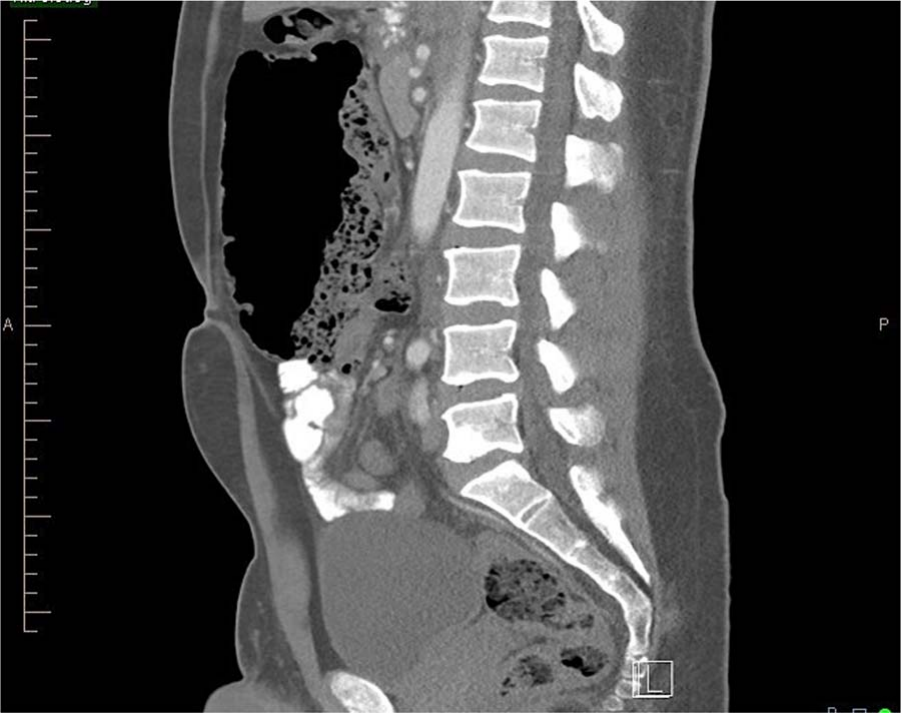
Sagittal view of CT abdomen showing higher and more anterior location of cecum; suggesting a hypermobile cecum (Normally the cecum is located lower in the right lower quadrant).
